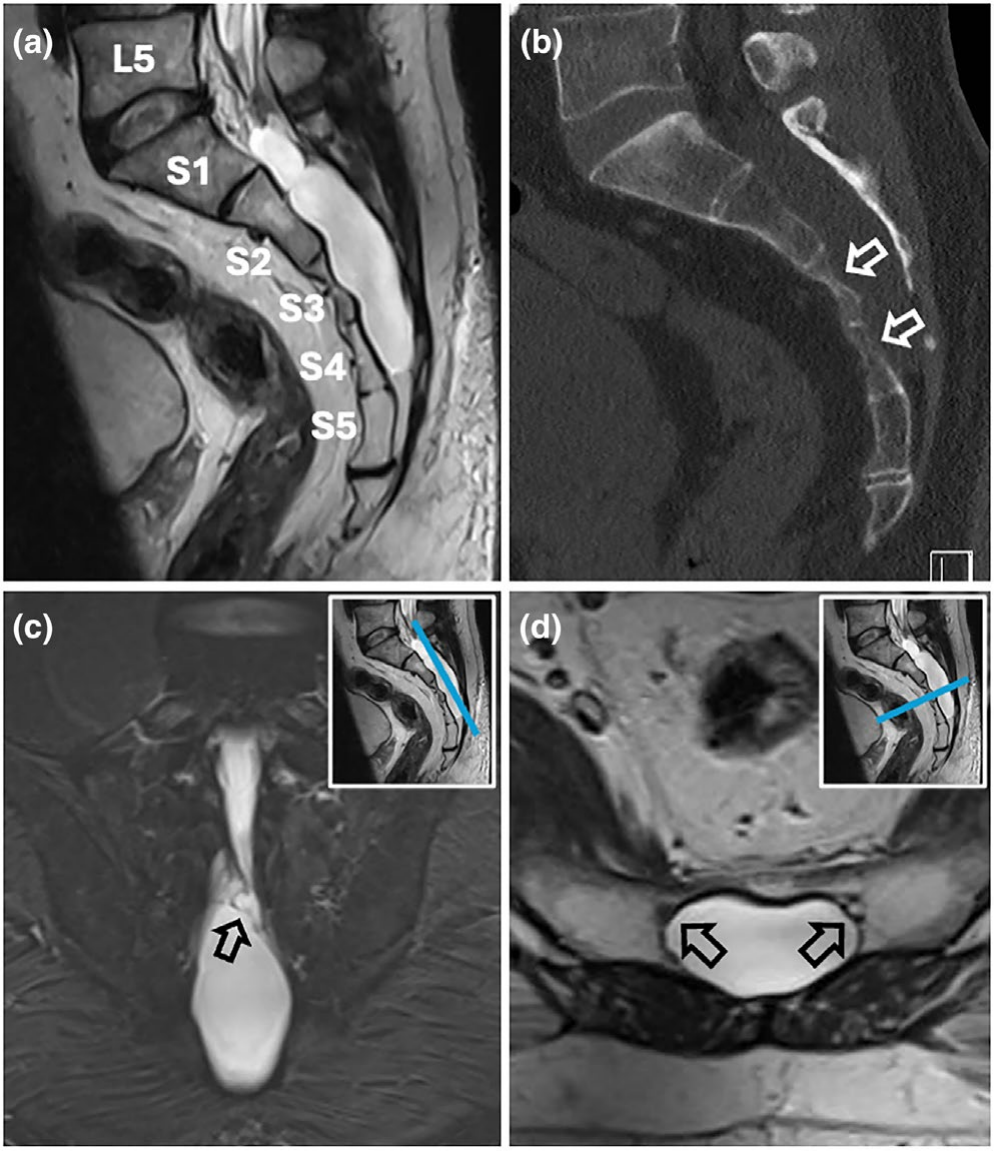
Imaging features of midline sacral meningeal cysts (MSMCs). (a) Sagittal T2‐weighted lumbosacral magnetic resonance imaging (MRI) slice showing an MSMC from lower S1 to the S4/S5 junction. (b) Sagittal lumbosacral computed tomography slice (bone window) demonstrating MSMC‐induced sacral scalloping (white arrows). (c) Oblique coronal T2‐weighted sacral MRI slice running through the MSMC's ostium (black arrow), seen to present with complex septations. Inset in right upper corner indicates level of the MRI slice. (d) Axial T2‐weighted sacral MRI slice through lower S3 level. Black arrows indicate S4 nerve roots pushed ventrally by the dorsally growing cyst.
